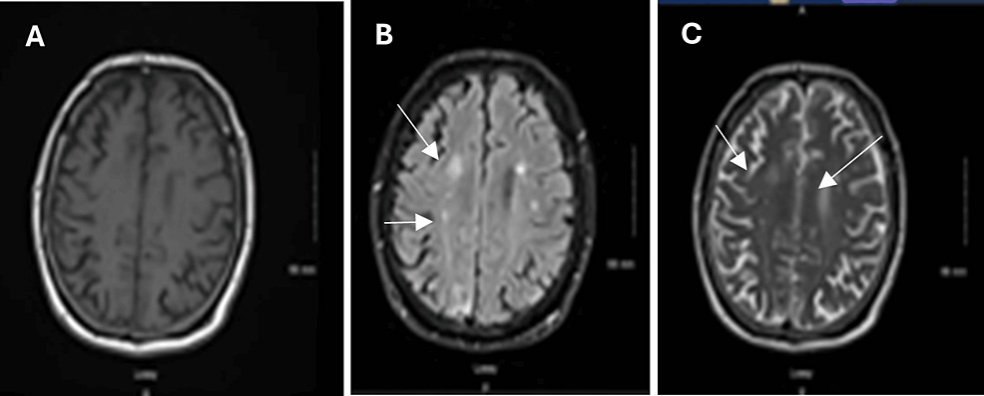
MRI with intravenous contrast shows a near-complete resolution of enhancement. Findings can be indicative of a response to therapy. Normalization of the diffusion signal is observed MRI: magnetic resonance imaging
